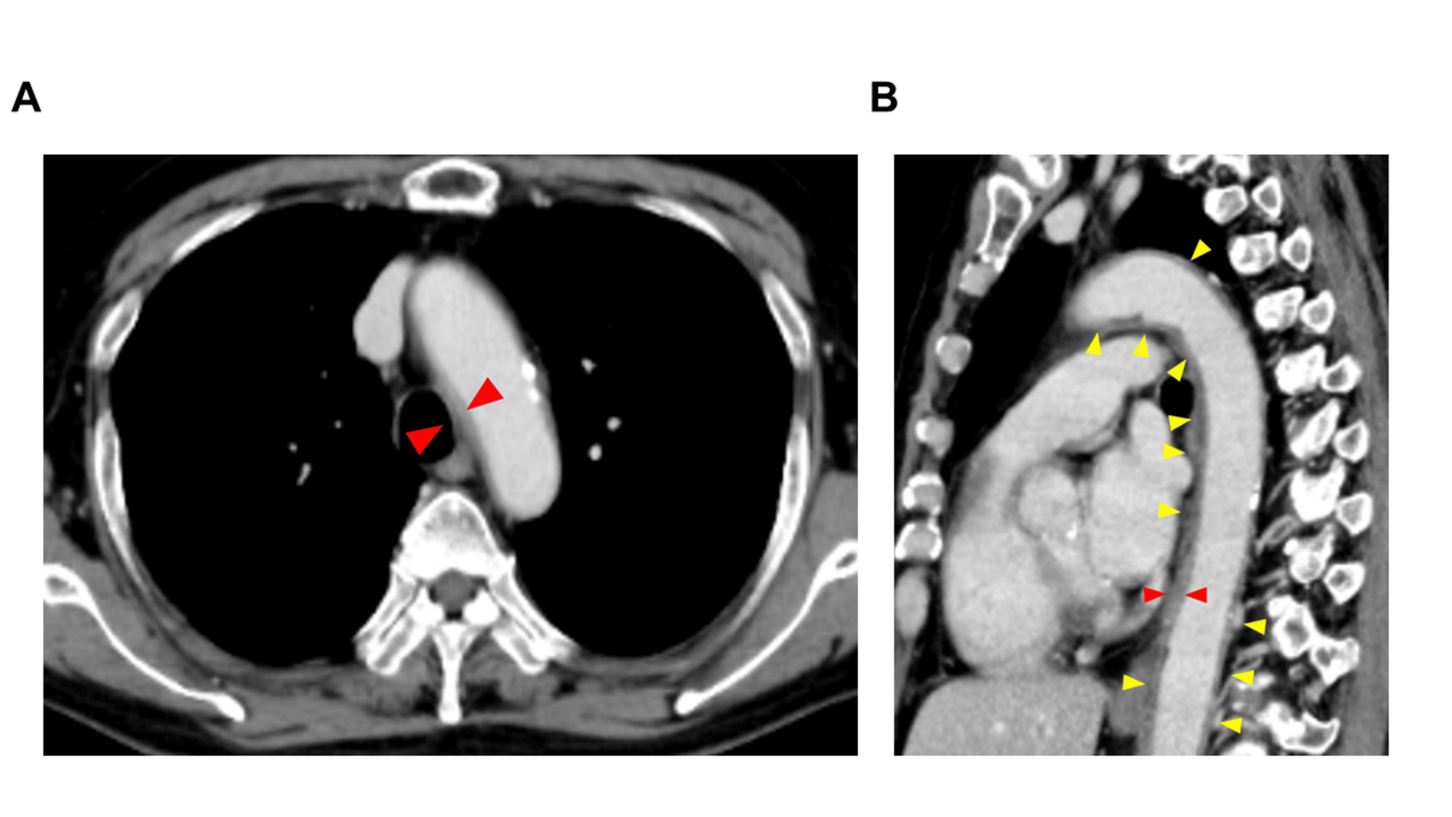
(A) Contrast-enhanced CT at diagnosis: a horizontal view. (B) Contrast-enhanced CT at diagnosis: a sagittal view. (A) This figure shows a horizontal view from a contrast-enhanced CT scan performed on day 19 after docetaxel initiation to identify the fever source, revealing extensive circumferential wall thickening from the common carotid artery to the aortic arch and abdominal aorta. (B) This figure displays a sagittal view. Yellow arrows indicate the extent of aortic inflammation at the time of aortitis diagnosis. Red arrows are used to compare the aortic wall thickness.
